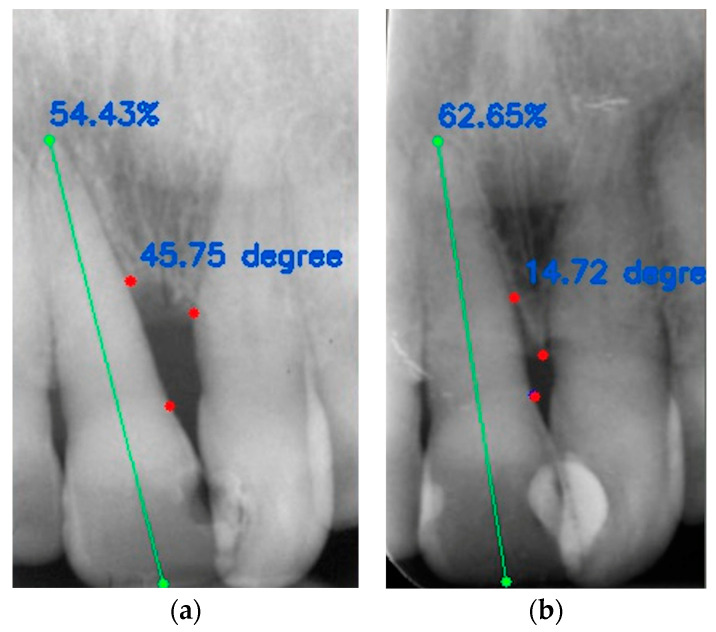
Radiographic case of a bone defect between the mesial surface of element 1.1 and the mesial surface of element 2.1: (a) angle at T0 is 45.75° and RSA at T0 is 54.43%; (b) angle at T1 is 14.72° and RSA at T1 is 62.65%. The green line represents the distance between coronal margin and root apex. The red dots represent the angle at the base of the intrabony defect, respectively at T0 and at T1.
